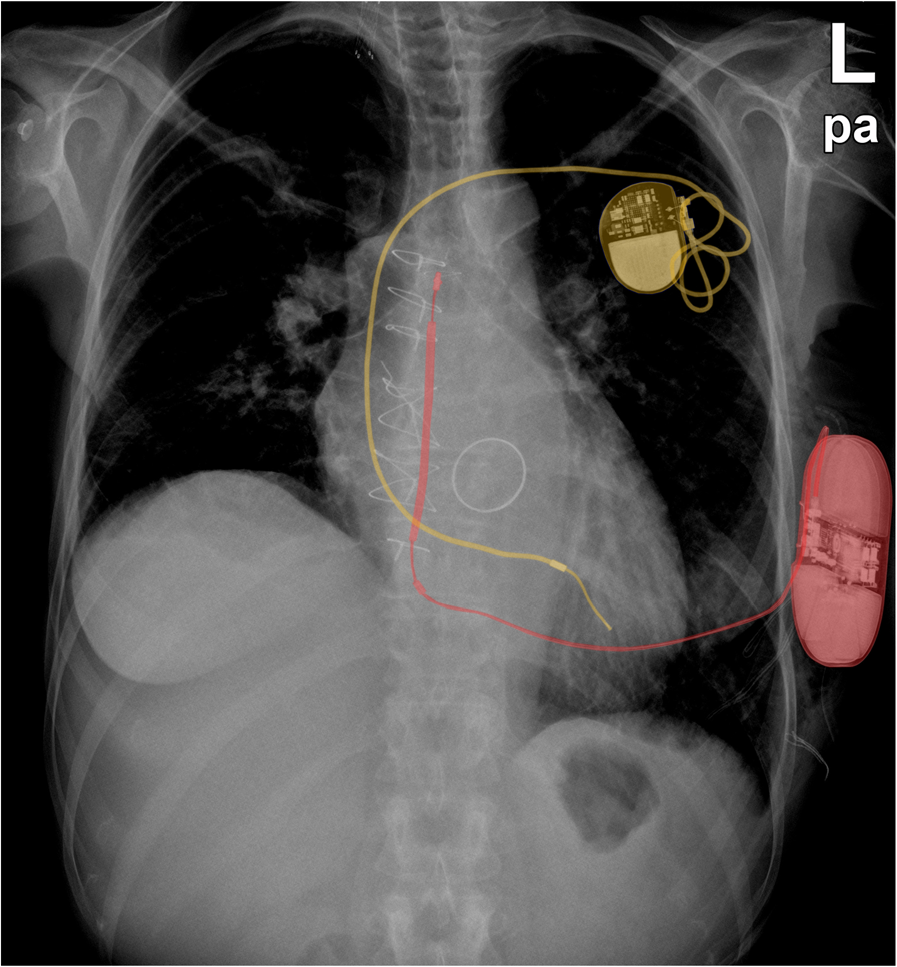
Chest x-ray of a patient carrying both, a conventional device (pacemaker (yellow)) and an S-ICD (red)
